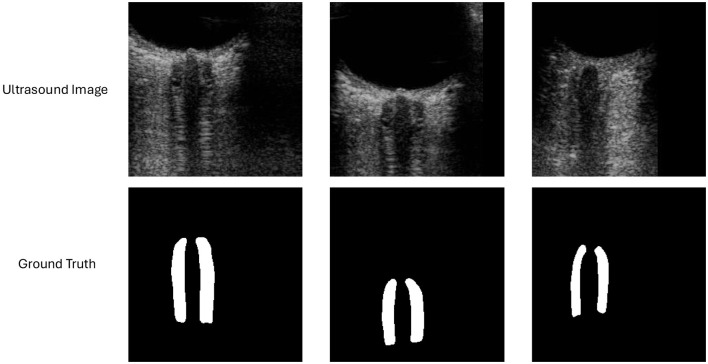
Sample ultrasound image for optic nerve segmentation and potential OND/ONSD quantification.
